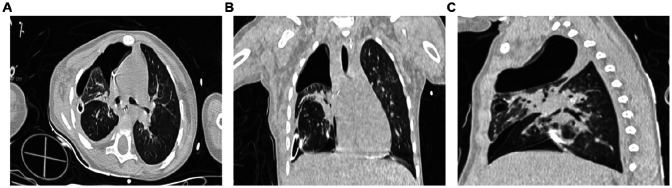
Chest CT scan on the first postoperative day. (A) Axial chest CT plain lung window; (B) Coronal chest CT plain lung window; (C) Sagittal chest CT plain lung window.
